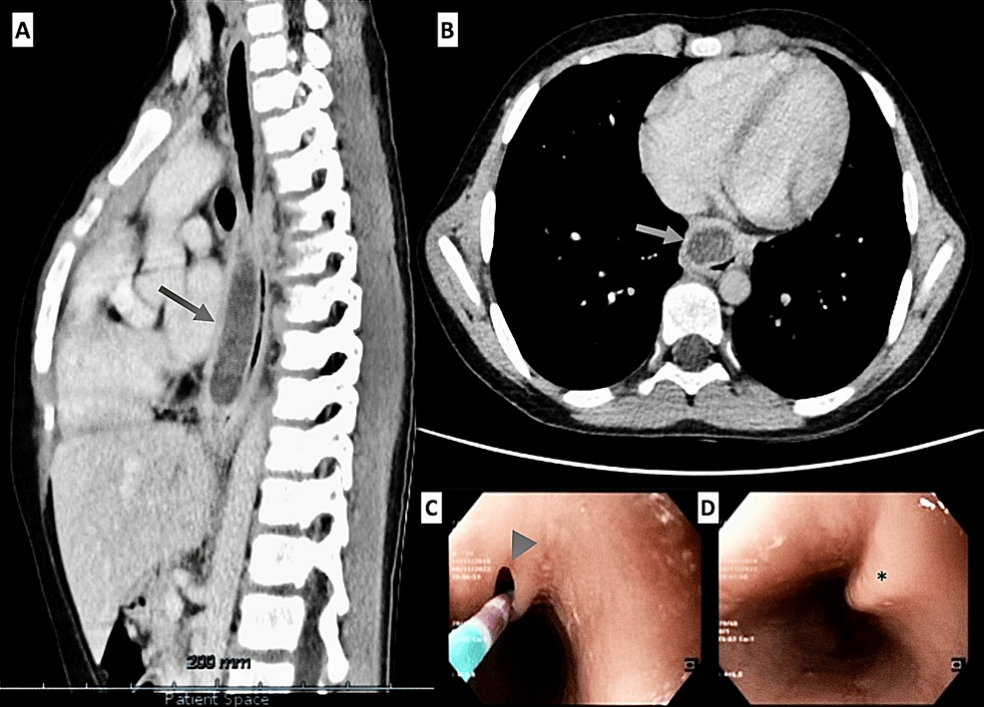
Imaging and endoscopic findings in tubular esophageal duplication Panels A and B: Thoracic CT showing a tubular formation with thick walls adjacent to the anterior thoracic esophagus, between the sixth and ninth thoracic vertebrae (arrow in panel A indicates the structure on a sagittal view, and arrow in panel B indicates the same formation on an axial view). The abnormality measures 6.5 cm longitudinally and 2.8 x 1.7 cm transversely, which was consistent with tubular esophageal duplication (TED) and mild upstream esophageal dilatation. Panels C and D: Upper digestive endoscopy revealing a well-preserved esophageal caliber with good distension. A 2 mm opening (arrowhead in panel C) at 21 cm from the dental arch spontaneously opened with insufflation. The remaining mucosa shows no lesions (asterisk in panel D).
